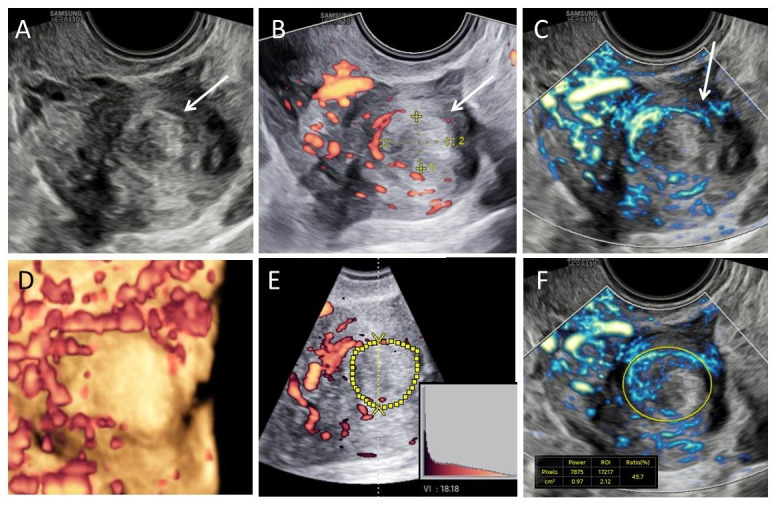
Representative images of a fibroid. A. Fibroid in B-mode; B. Fibroid in power Doppler mode; C. Fibroid in MV-flow^TM^ mode. Arrow indicates circumferential flow; D. 3D power Doppler reconstruction fibroid; E. How to determine the vascular index in 3D power Doppler mode, yellow circle depicts the fibroid as region-of-interest; F. How to determine the vascular index in MV-flow^TM^ mode.
